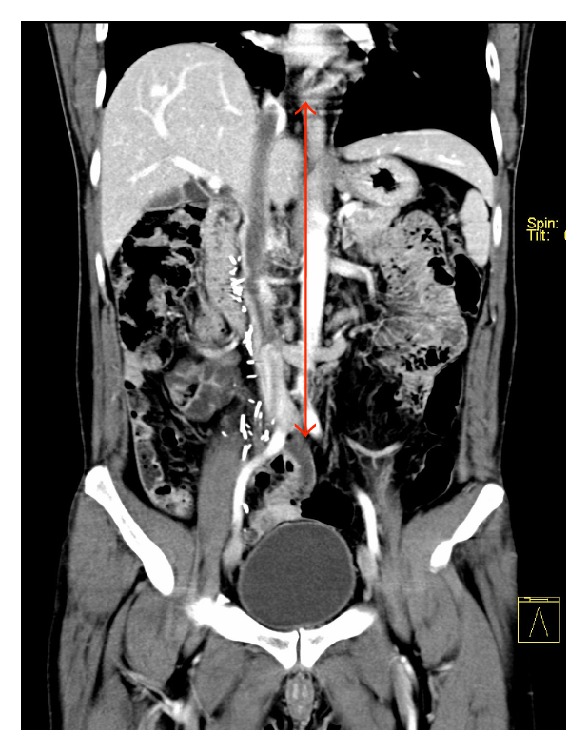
Reformatted coronal CT image demonstrating contiguous tumour thrombus in the inferior vena cava extending to the junction of the right atrium.
